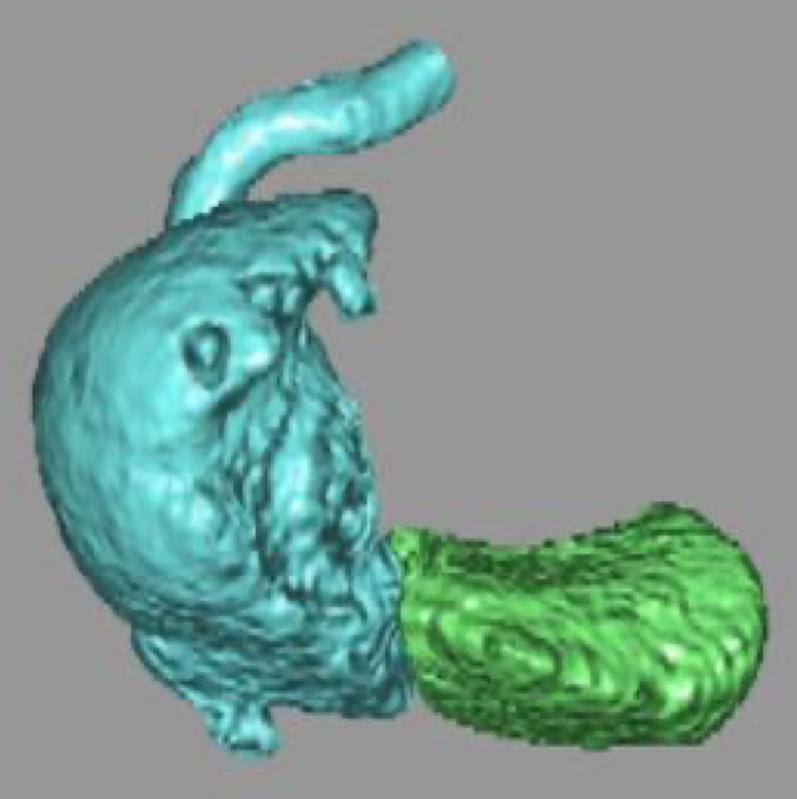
Postnatal 3-Dimensional Cardiac Computed Tomography Displaying Coronary Sinus Aneurysm (Green) and Dilated Right Atrium (Blue)
